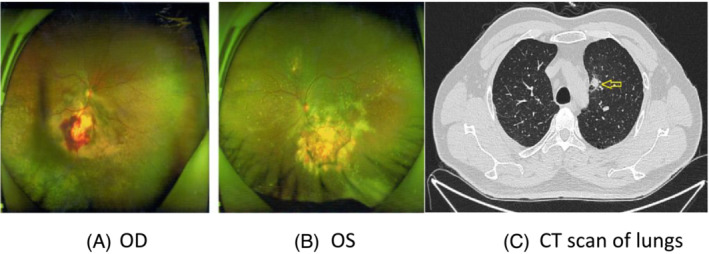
Eye fundus examination and CT scan of lung in March 28, 2021. Ophthalmofundoscopy showed that bilateral choroidal lesions were significantly improved with (A, B) a little bleeding and exudation in the choroids. The CT scan of the lung showed that the nodule in left upper lung significantly shrank (C).
